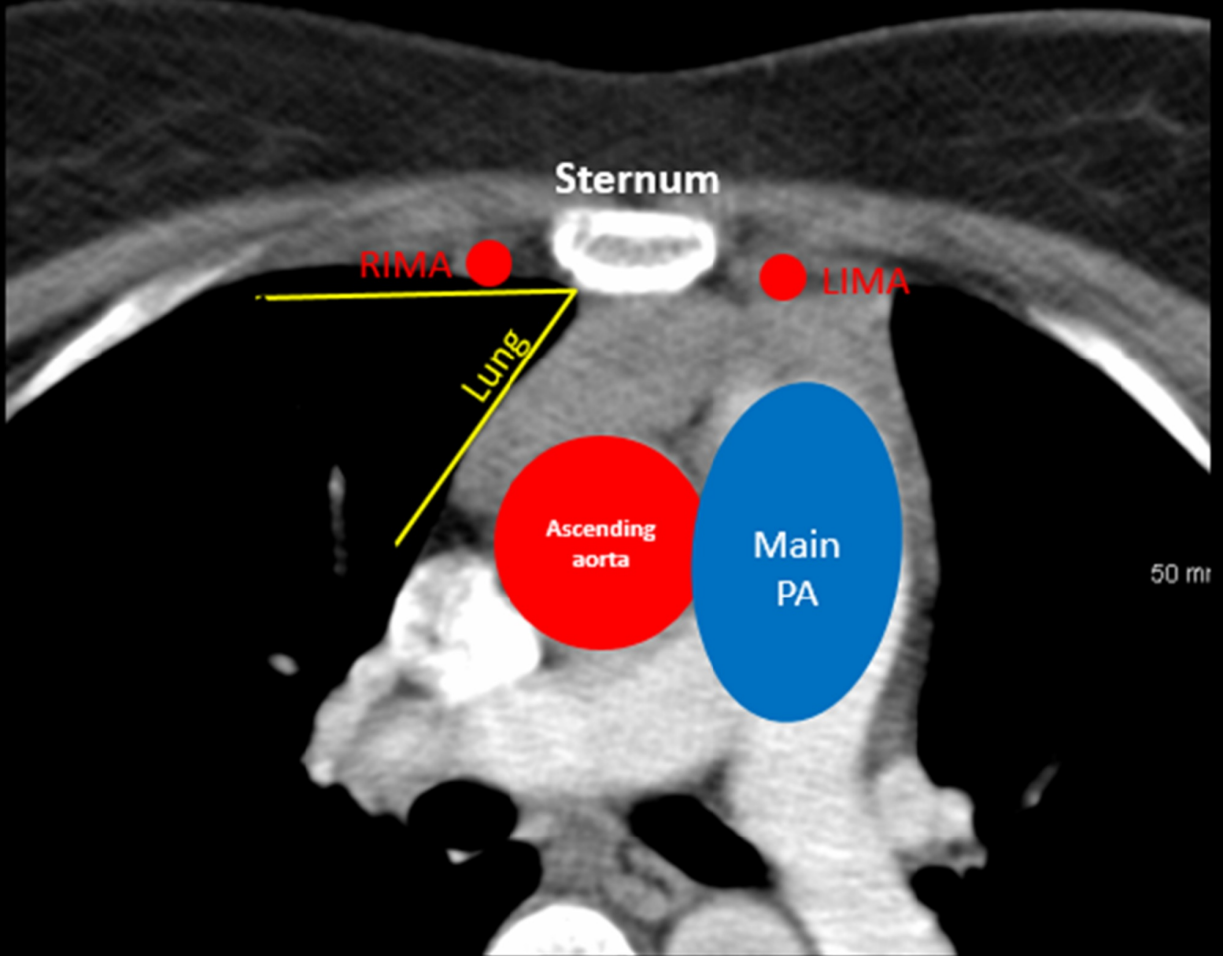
Anatomical Depiction of Vital Structures Surrounding an Anterior Mediastinal Mass The computed tomography of the chest shows the difficulty the interventional operator would have when attempting to biopsy the anterior mediastinal mass. The sternum is the most anterior structure to the mediastinal mass. Lateral to the sternum on both sides are the left (LIMA) and right (RIMA) internal mammary arteries (smaller red dots), and they are both anterior to the mediastinal mass. The lungs are shown bilaterally, represented by the black areas, with the border of the right lung shown. Both lungs are lateral to the mediastinal mass. The main pulmonary artery (PA) and ascending aorta are posterior to the anterior mediastinal mass.
